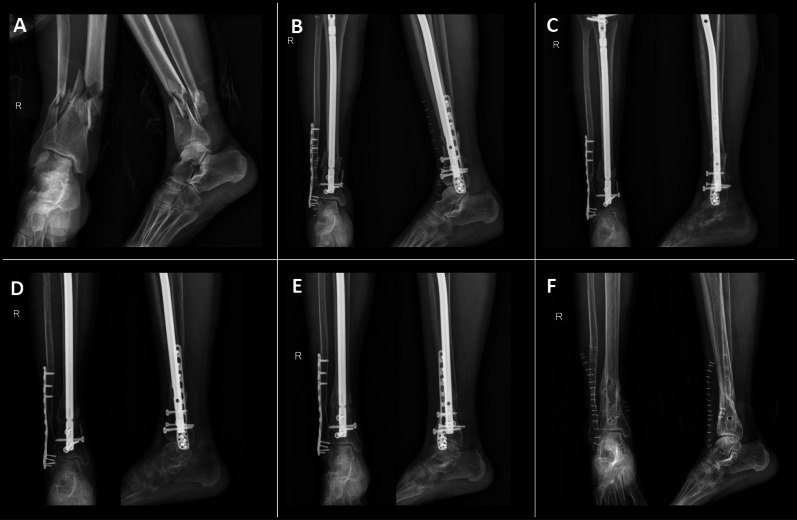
Patient with UDTF treated with IMN: A Right-sided ultra-distal tibia and fibula fractures; B Postoperative radiograph at 1 day; C Radiograph at 3 months postoperatively; D Radiograph at 6 months postoperatively; E Radiograph at 1 year postoperatively; F Radiograph following implant removal
